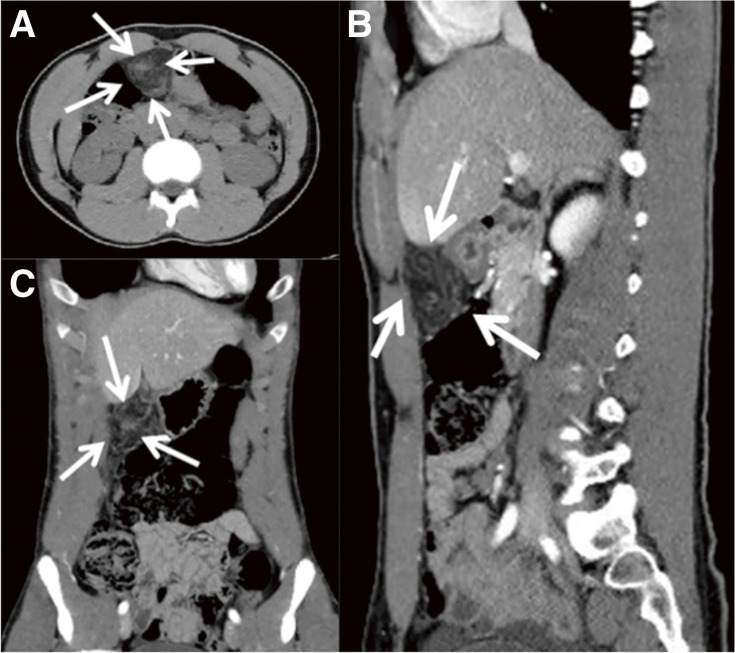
CT results of Case 1. (A) Transverse plane. (B) Sagittal plane. (C) Coronal plane. Whirl sign of omental torsion and increased fat concentration on CT (white arrow). CT, computed tomography
